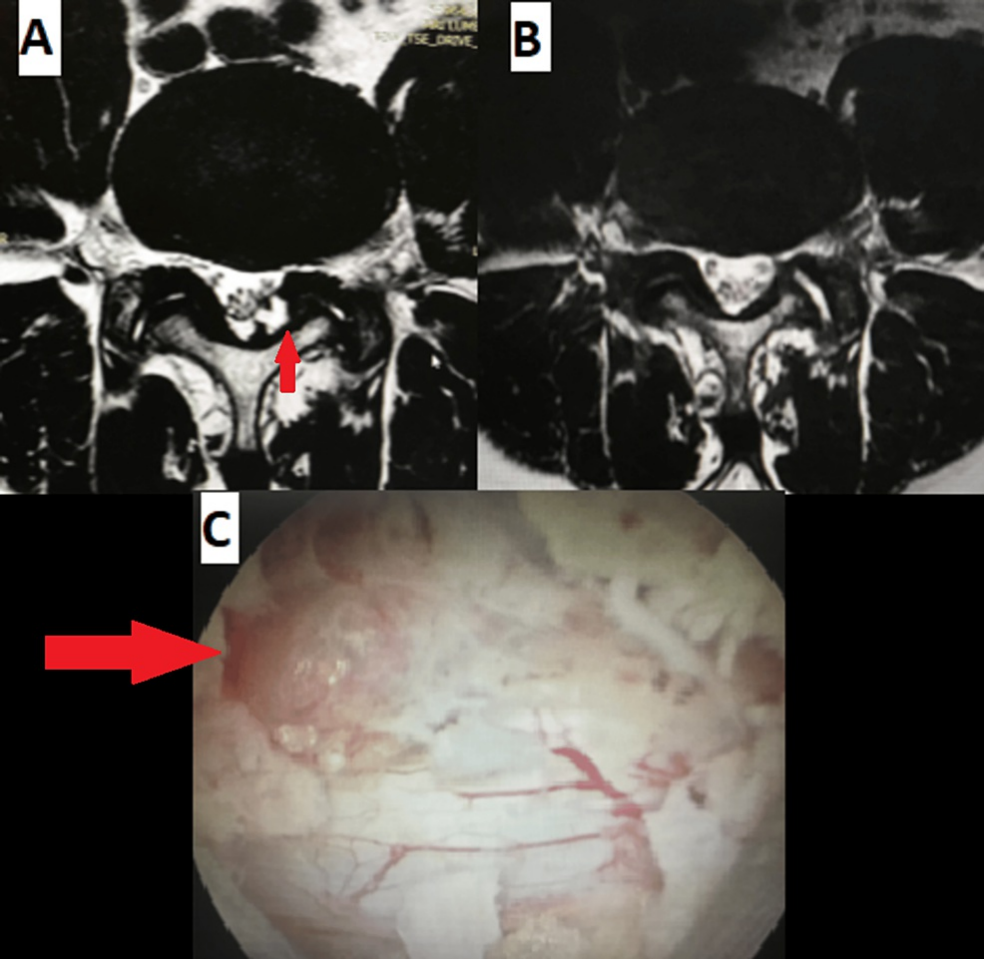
(A) Pre-operative showing ligamentum flavum cyst (red arrow) and (B) Post-operative axial MRI axial images with (C) an intraoperative endoscopic picture showing ligamentum flavum cyst (red arrow) who was treated successfully with full endoscopic interlaminar technique.
